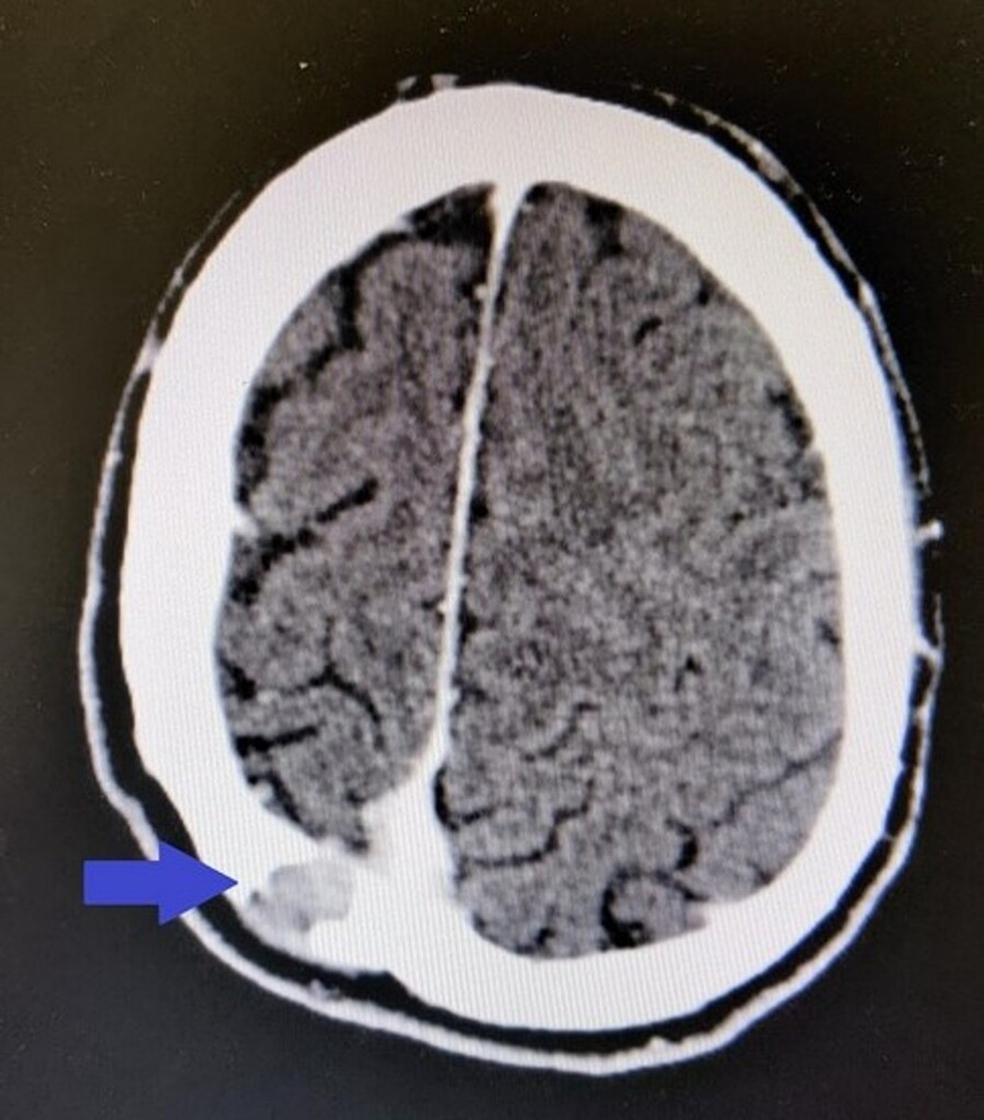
A CT scan showing an osteolytic damage to the skull occipitally (blue arrow).
